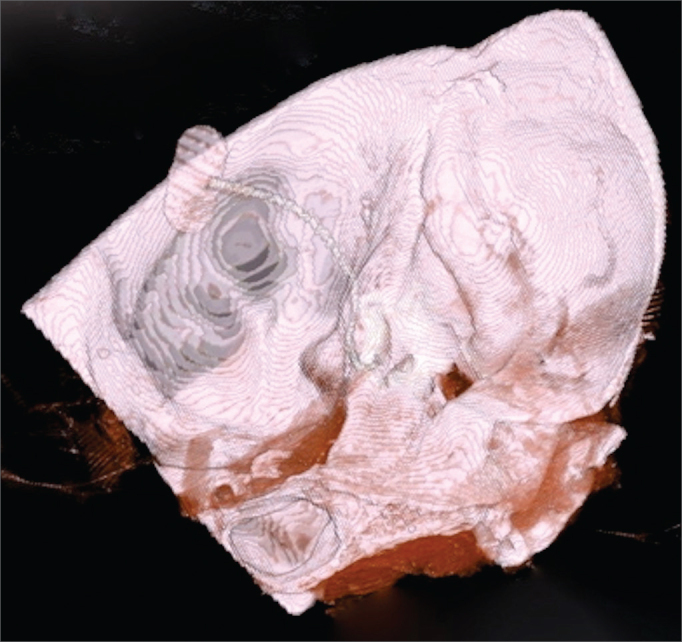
Right temporal bone high-resolution CT scan. 3D reconstruction showing the placement of the CI array through the middle cranial fossa.
